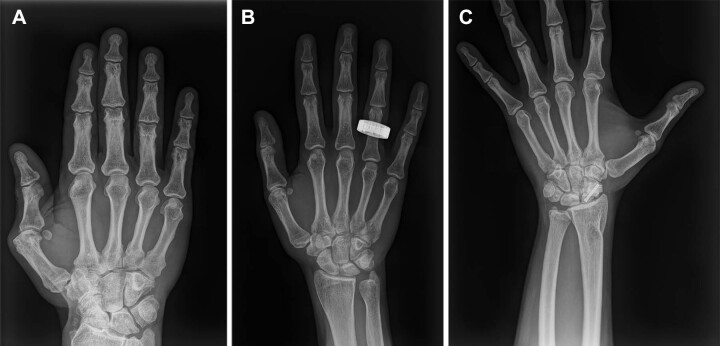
Representative preflight, in-flight, and postflight hand radiographs.
